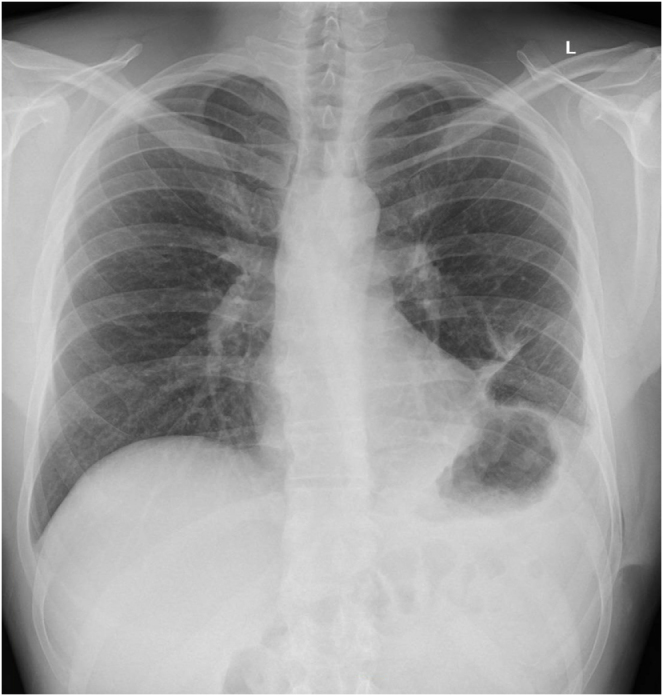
Postoperative chest radiograph following chest drain removal.
